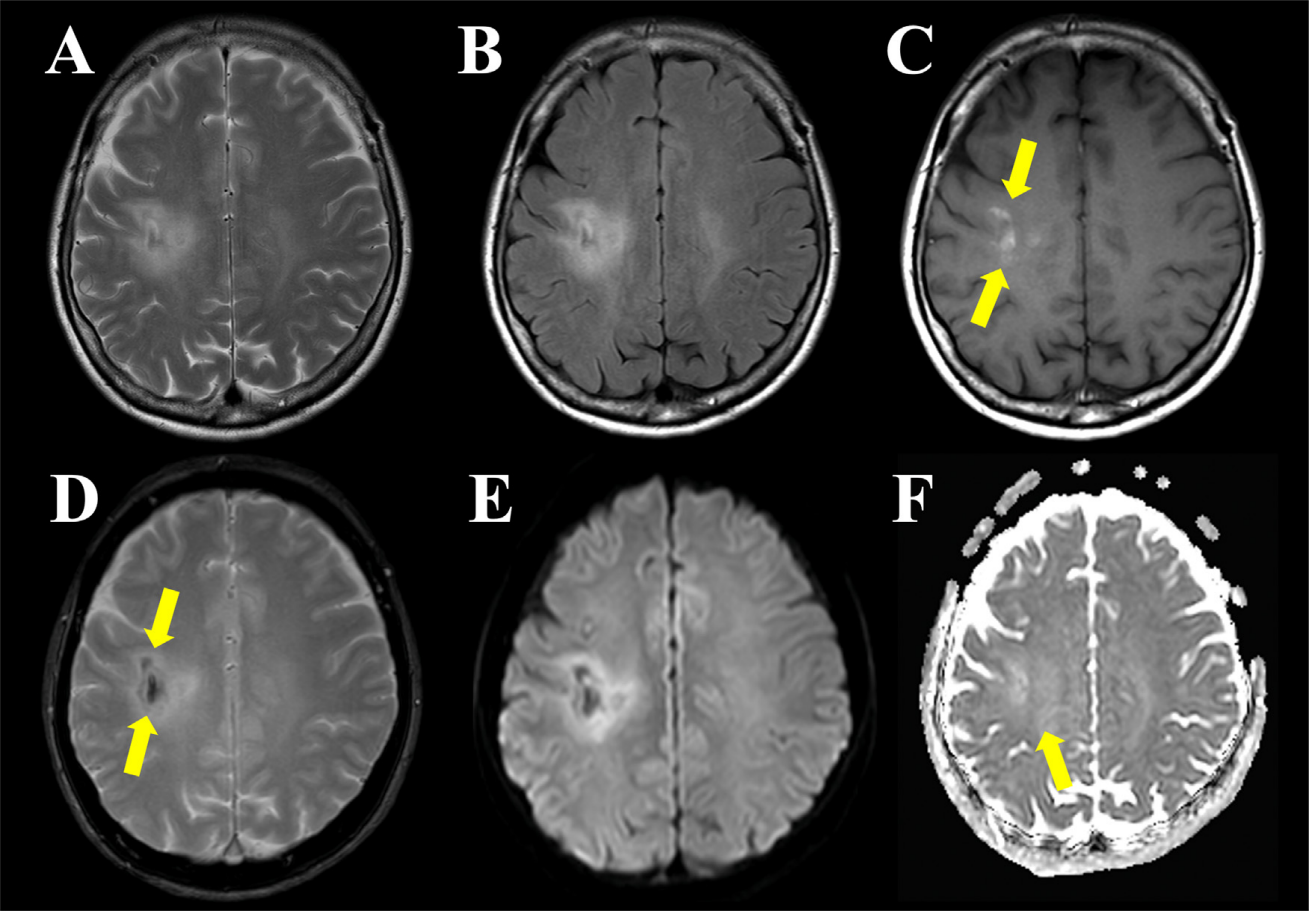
Brain MRI findings. Brain MRI with T2-weighted (A) and FLAIR (B) imaging shows an ill-defined area of high signal intensity extending across the right frontal and parietal lobes. In addition, faint hyperintensity on T1-weighted imaging (C, arrow) and hypointensity on T2*-weighted imaging (D, arrow) in the deep white matter of the right frontal and parietal lobes suggest the presence of calcifications. Diffusion-weighted imaging (E) shows hyperintensity in the deep white matter of the right frontal and parietal lobes, while the apparent diffusion coefficient (ADC) values are comparable to those of the contralateral normal white matter (F, a mean ADC value of 0.83 × 10⁻³ mm²/s in the area indicated by the arrow).
